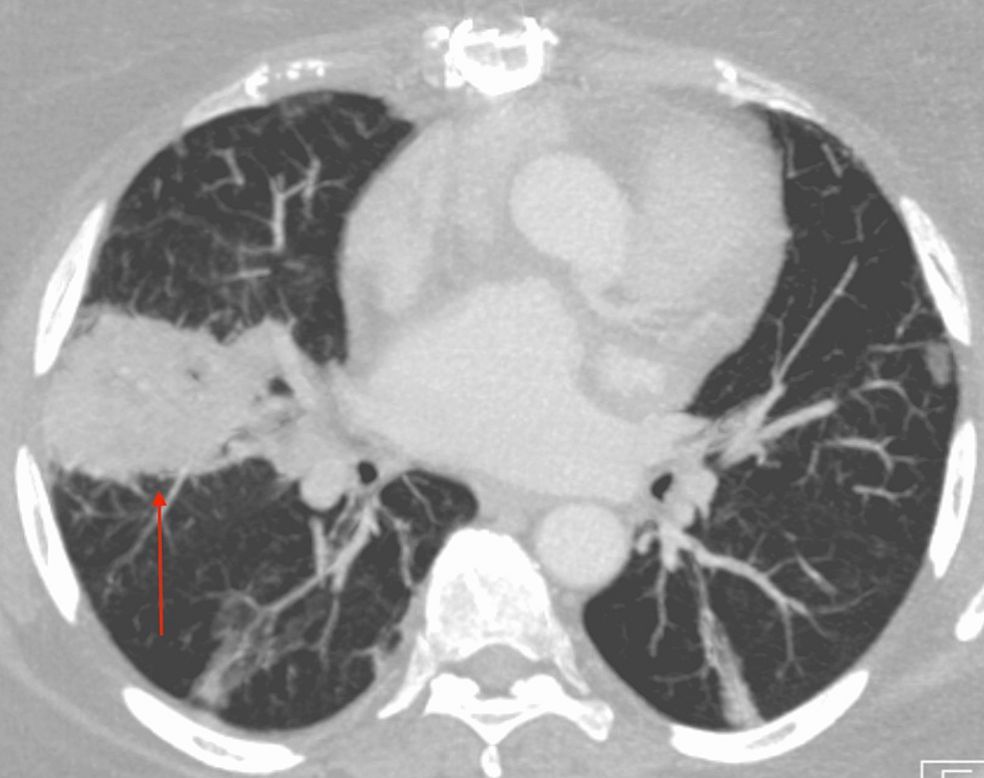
CT thorax The red arrow is pointing at the right middle lobe cavitary lesion CT: computed tomography
